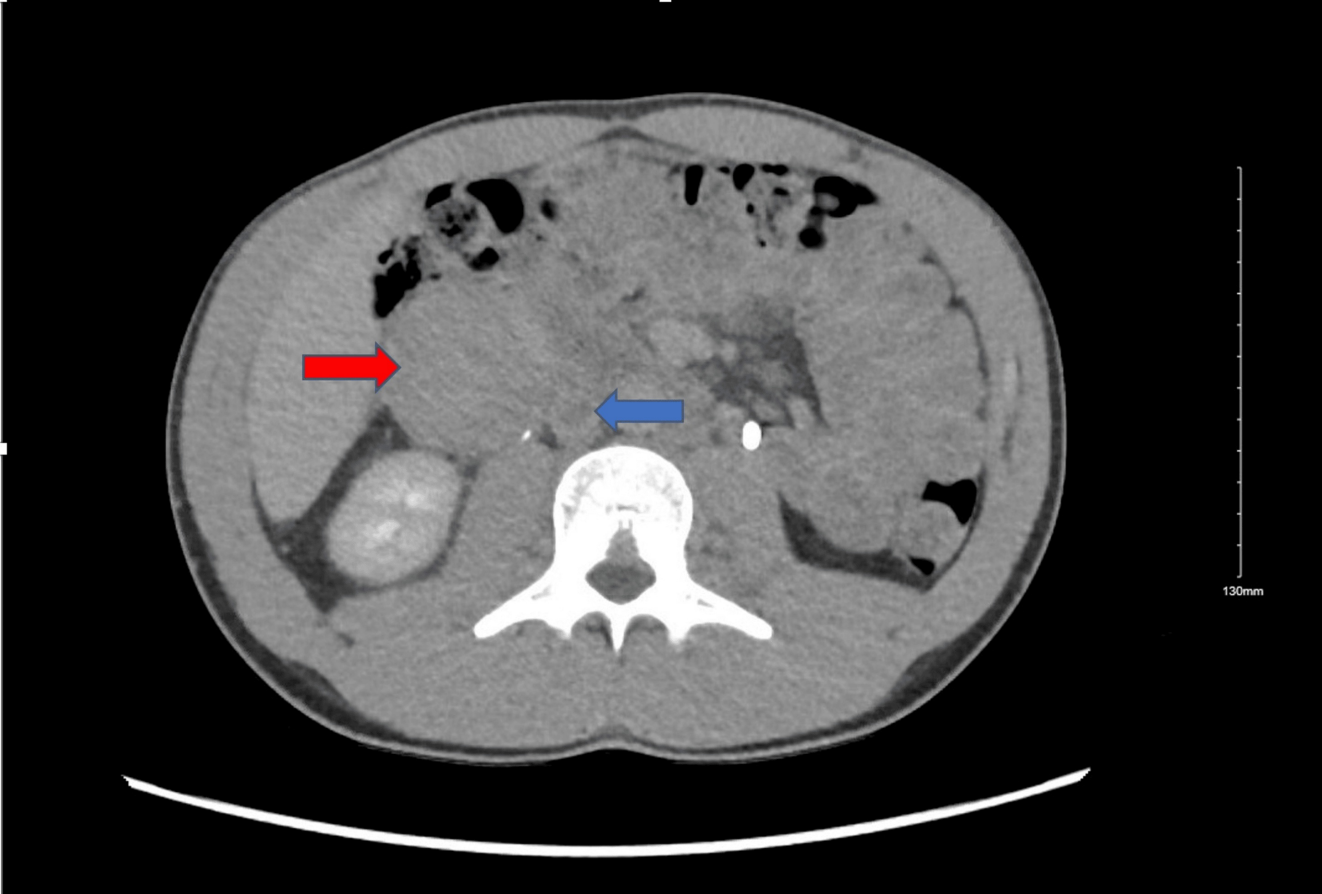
CT of the abdomen demonstrating a large right retroperitoneal mass measuring 7.5 cm (red arrow) and venous thrombus throughout the infrarenal inferior vena cava (blue arrow).
